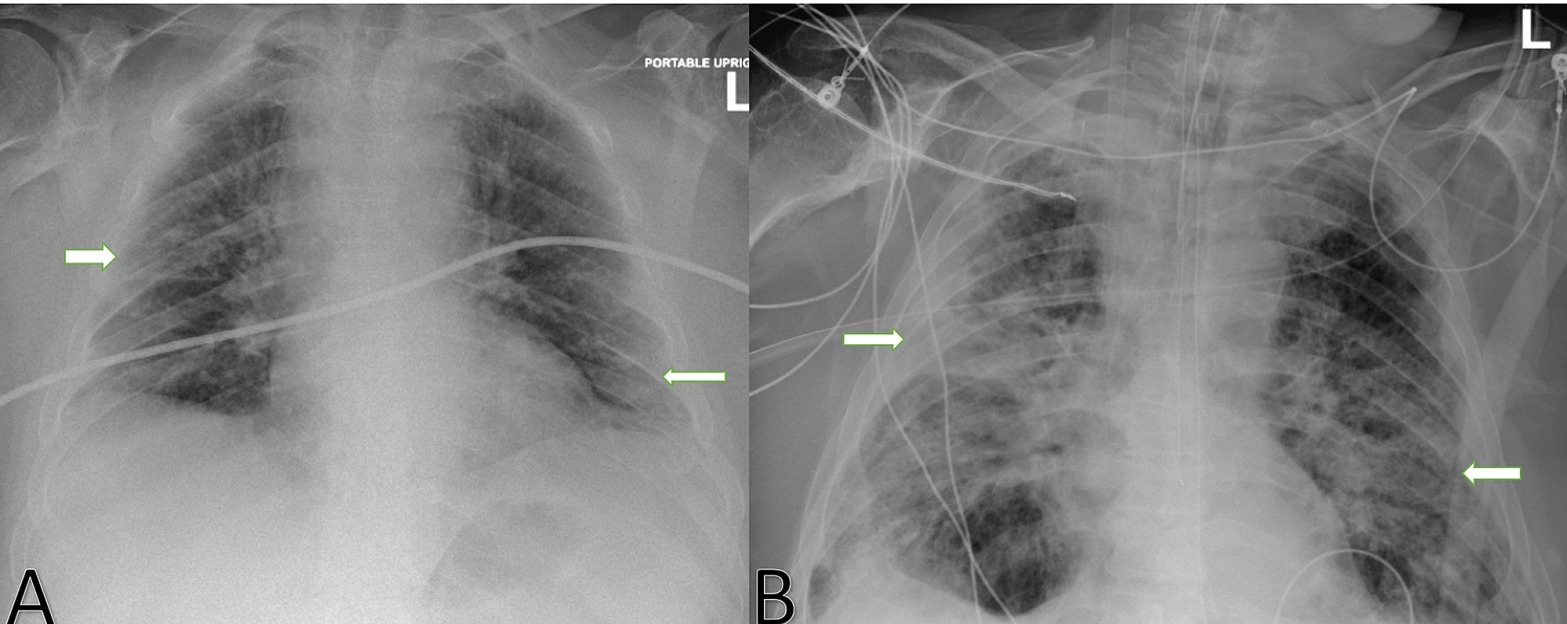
Posterior-anterior view of chest X-ray (CXR) at admission (A) and at the diagnosis of invasive aspergillosis (B) CXR on the left (A) shows bilateral patchy infiltrates as marked by white arrows, while CXR on the right (B) shows dense multilobar patchy consolidations marked by white arrows.
